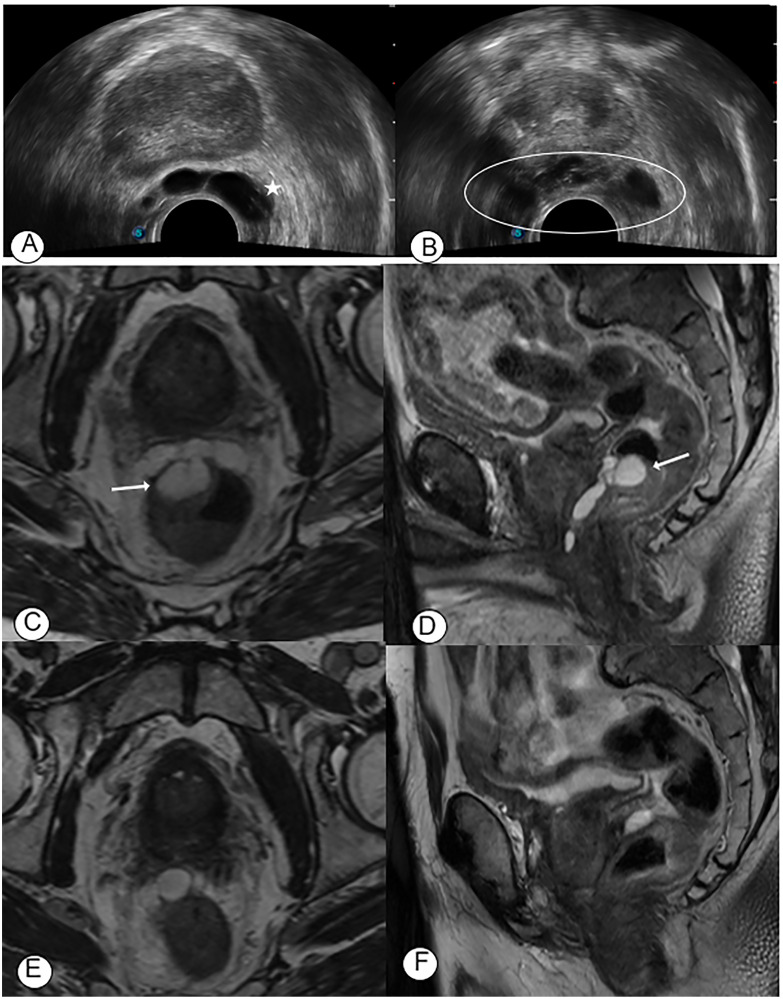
(A) Ultrasound imaging at the time of the procedure showing spacer gel infiltrating into the rectal wall (star). (B) Ultrasound imaging after hyaluronidase was used showing the resolution of rectal infiltration (circle). (C, D) Diagnostic MRI scan showing rectal wall infiltration (white arrow). (E, F) Treatment planning MRI scan showing resolution of rectal wall infiltration after hyaluronidase injection. *Abbreviation:* MRI = magnetic resonance imaging.
